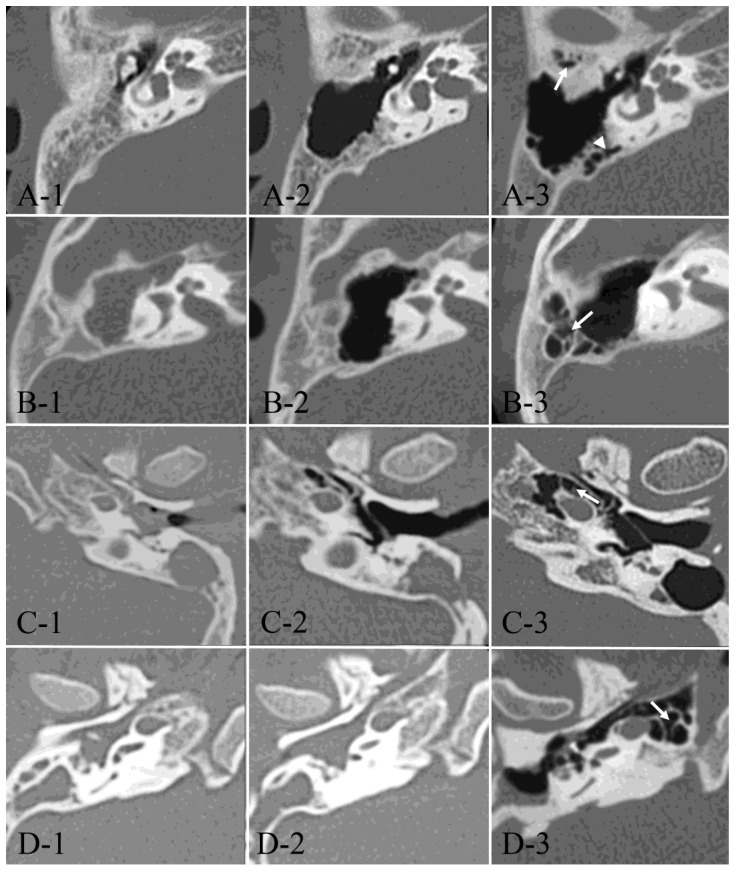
Developmental process of postoperative pneumatization. Axial CT images from four typical cases (A–D) at preoperative, one year postoperative, and the last follow-up examinations (1–3). (A-1–A-3): A 9-year-old male with right-sided AC showed postoperative development of the anterolateral area of periantral cells (arrow) and the medial parts of periantral cells (arrowhead). At the age of 14. (B-1–B-3): A 5-year-old female with right-sided AC showed postoperative development of the posterolateral area of periantral cells (arrow) at the age of 13. (C-1–C-3): A 6-year-old male with left-sided AC showed postoperative development of peritubal cells (arrow) at the age of 22. (D-1–D-3): A 5-year-old male with right-sided AC showed postoperative development of petrous apex cells (arrow) at the age of 24.
